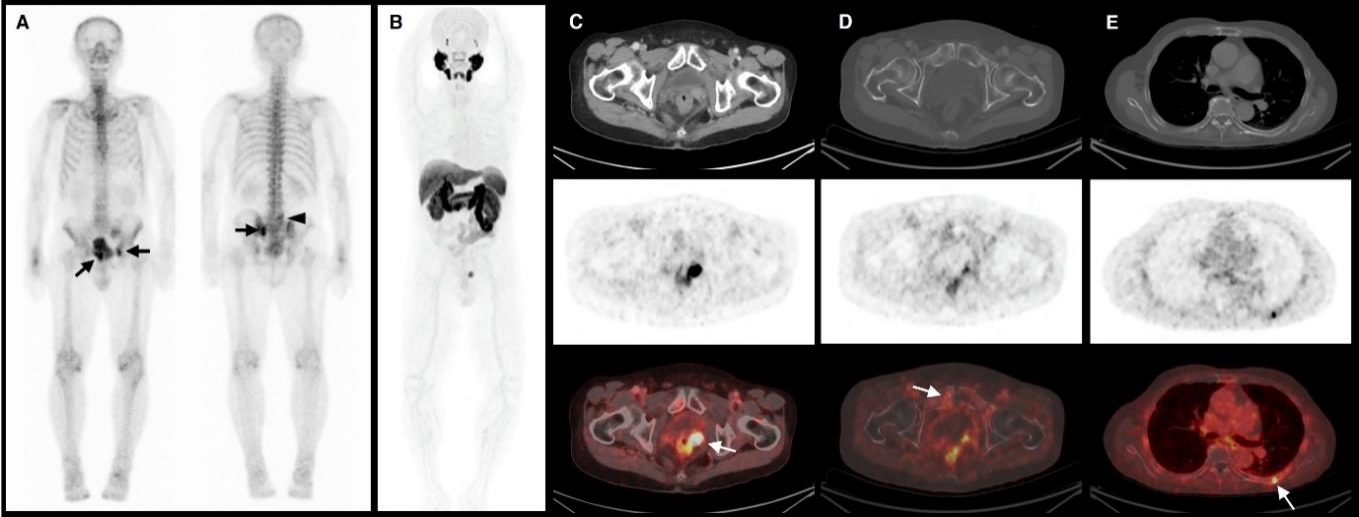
A 75-year-old patient (Gleason score 9(4+5), pT3aN0M0) with BCR (tPSA=1.78 ng/mL) almost 7 years after robotic-assisted laparoscopic radical prostatectomy. (A) Bone scan showed increased uptake at the right superior pubic ramus, left sacroiliac region, and left acetabulum (black arrows) that were most likely bone metastasis. Another area of increased uptake at the left side of L5 vertebra was likely due to degenerative change (black arrow head). (B) ^18^F-PSMA-1007 PET/CT scan performed on the same patient revealed (C) local recurrence at the prostate bed, (D) mildly PSMA-avid osteoblastic lesions at the right superior pubic ramus, (not shown) left sacroiliac region, and left acetabulum, that were consistent with bone scan findings and supported a diagnosis of bone metastasis. (E) Focal PSMA avidity at the left 7^th^ rib without CT correlate favored non-specific bone uptake
